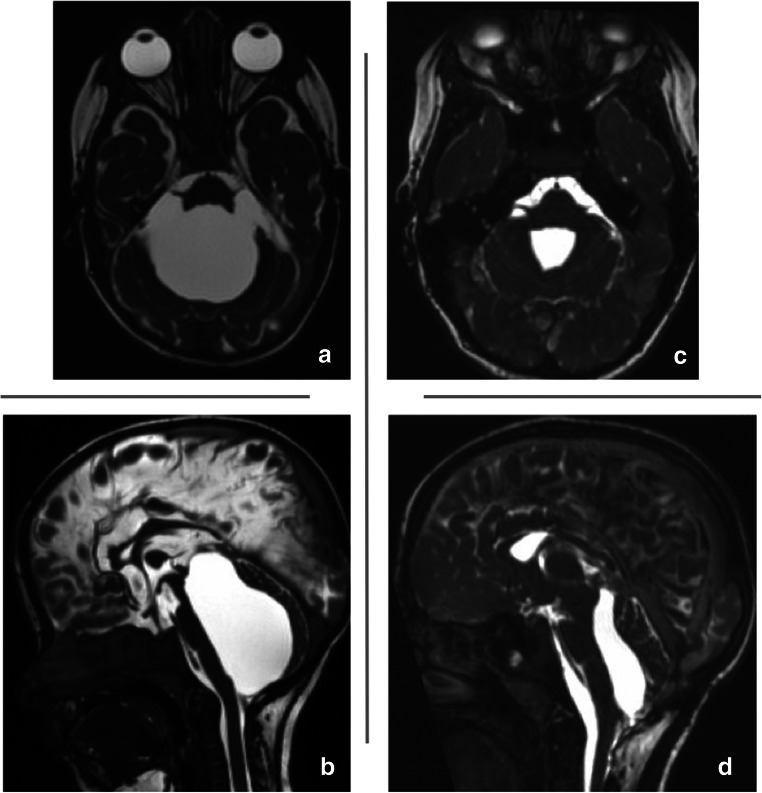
MR images demonstrating a TFV in a preterm with PHH, came at age of 5 years for a regular follow-up. a, b Axial and sagittal MR T2-weighted images showing ballooning of fourth ventricle with massive brainstem compression. c, d Axial T2-weighted images 5 years after failed cranio-cervical decompression and insertion of fourth ventricular catheter and connection to implanted VP shunt
